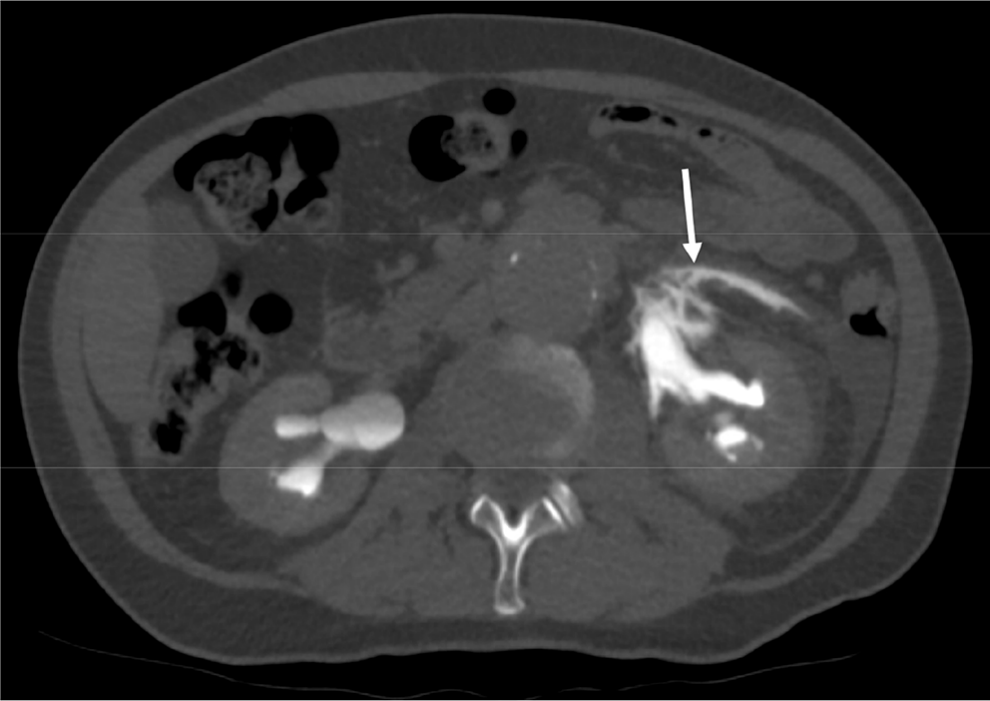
A patient with blow-out of the renal pelvis due to retroperitoneal fibrosis. An excretory phase at CT shows leakage of contrast from the renal pelvis into the perirenal space and also extending through the ARF into the prerenal space (arrow). The focal discontinuation of the fascia is most likely pathological due to the presence of the retroperitoneal fibrosis
